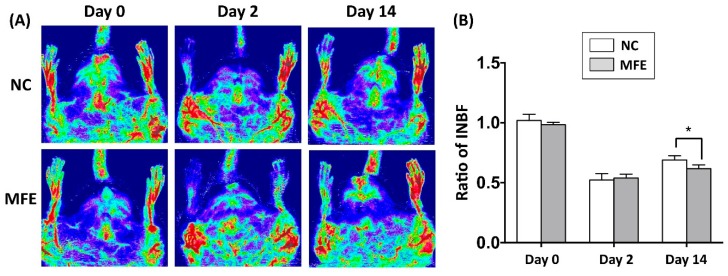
Maternal fructose exposure reduced blood flow recovery after induction of critical limb ischemia. (A) Laser Doppler scanning to determine the blood flow recovery after induction of CLI. (B) Quantitation and calculation of blood flow. The parameter ratio of ischemia to normal blood flow (INBF) was used to evaluate the blood flow recovery initiated by neovascularization after CLI. On day 2 after CLI and day 0 prior to CLI, the ratio of INBF showed no difference between NC and MFE groups. However, by day 14, the blood flow recovery in the NC group was significantly higher than the MFE group. NC, normal control; MFE, maternal fructose exposure; CLI, critical limb ischemia. Error bars represent the standard deviation (SD). * Indicates statistical significance between NC and MFE group. *n* = 8 for each group.
